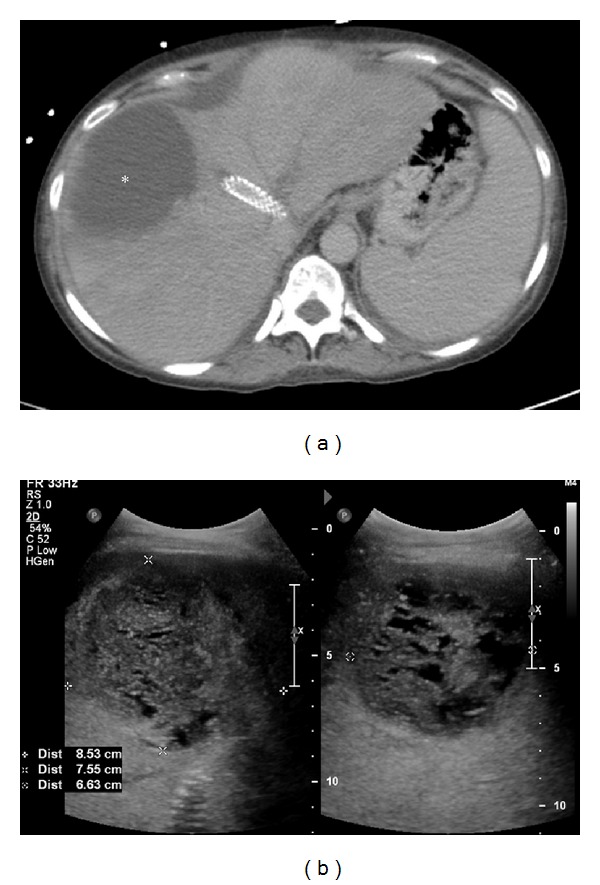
(a) Axial contrast-enhanced CT of the upper abdomen shows a well-defined nonenhancing hypodense area liquefaction necrosis of infarcted liver parenchyma in hepatic segments V and VIII (*asterisk*). Again noted is the hypodense material within the left hepatic vein, consistent with hepatic vein thrombosis. (b) Ultrasound of the right lobe of the liver demonstrates a heterogeneous collection that corresponds to hepatic infarction.
